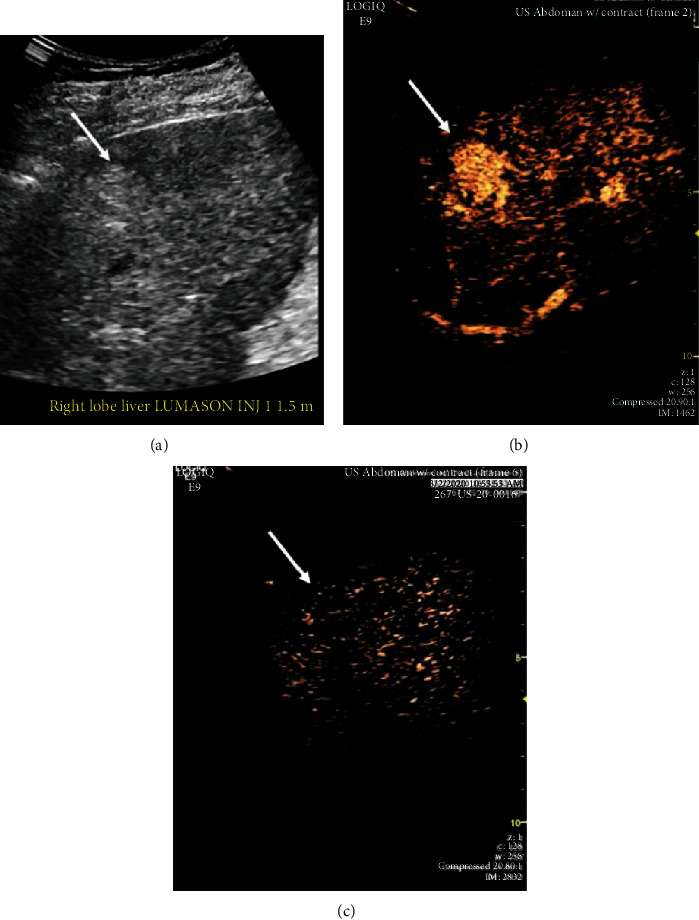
Contrast-enhanced ultrasound (US) demonstrates a 2.5 cm mass in segment 7 (arrow) that is echogenic on grayscale (a) and has early enhancement relative to the liver parenchyma (b). At 5 minutes postcontrast injection, there is a very subtle washout (c).
